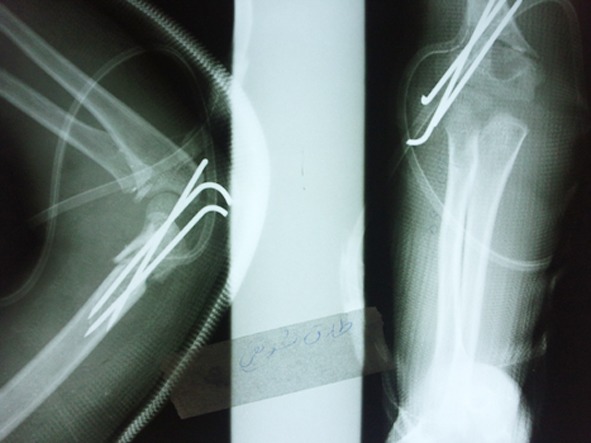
Post op. elbow radiography.
